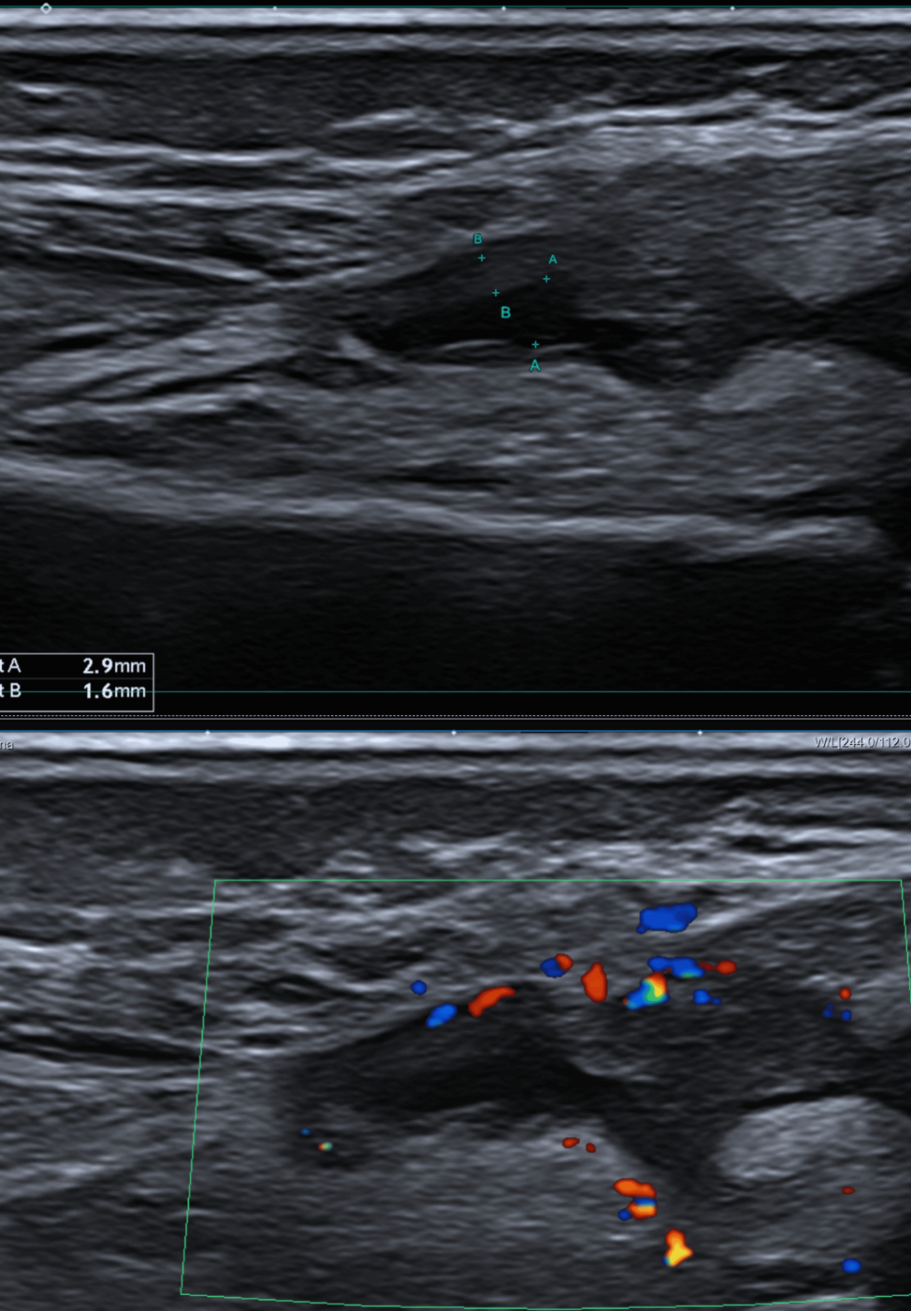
Ultrasound of the left knee
